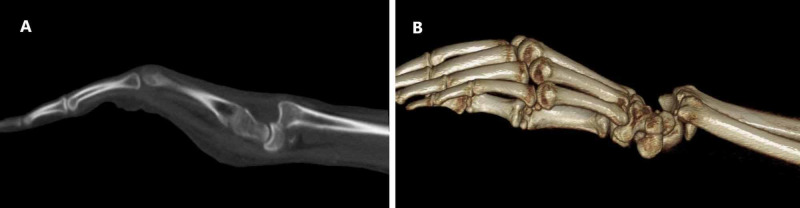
Preoperative CT images of the left wrist. A) Standard CT image; B) 3D CT image
